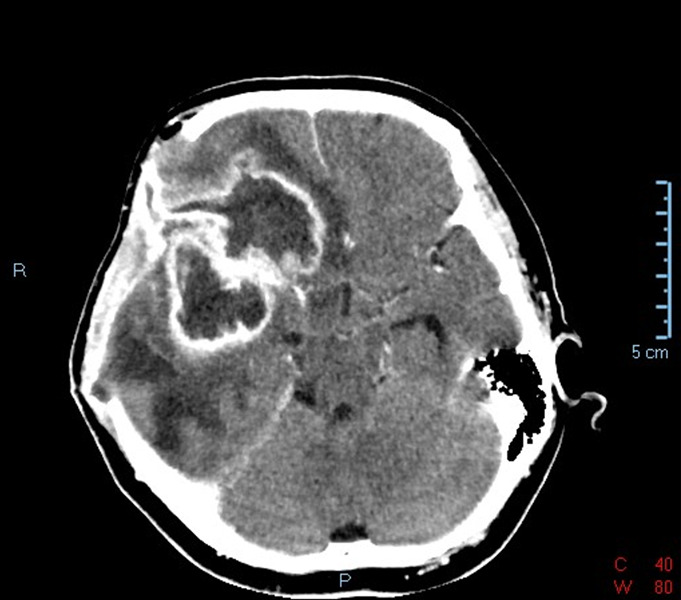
The cerebral CT done during the second admission It shows enlargement of the remaining tumor postoperatively and reduced inflammation.
